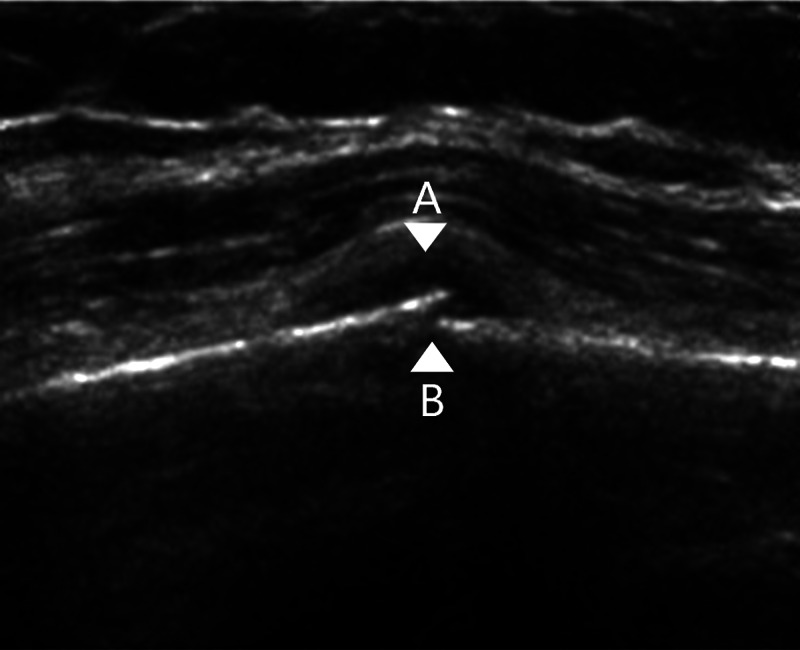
Ultrasound of a diaphyseal tibial fracture demonstrating a break in the cortex, periosteal haemtoma formation and periosteal lift. Ultrasound images from Qadi et al., 2020 [9] A- Periosteal haematoma B- Loss of continuity of the bony cortex and periosteal lift Original images authorised by respective owners
